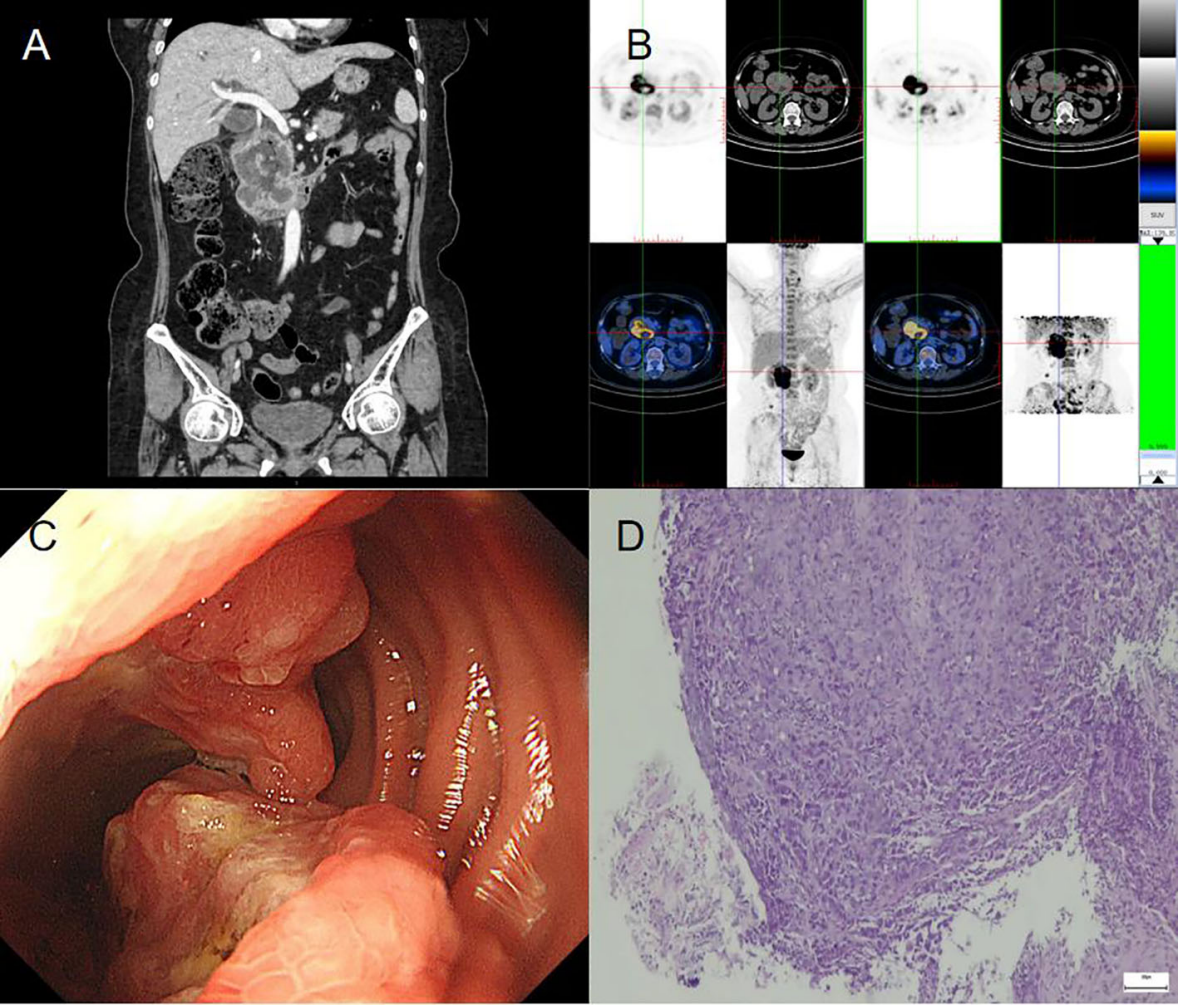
CT scan of the abdomen revealed a 4×5cm tumor in the descending duodenum (A). Radionuclide imaging indicated the intestinal wall of descending duodenum thickening and increased glucose metabolism (B). An electronic gastroscopy examination revealed a substantial tumor in the descending segment of the duodenum (C). Biopsy results confirmed a poorly differentiated malignant duodenal tumor and showed epithelial differentiation characteristics consistent with the metastasis of poorly differentiated carcinomatous component in endometrial carcinosarcoma (D).
